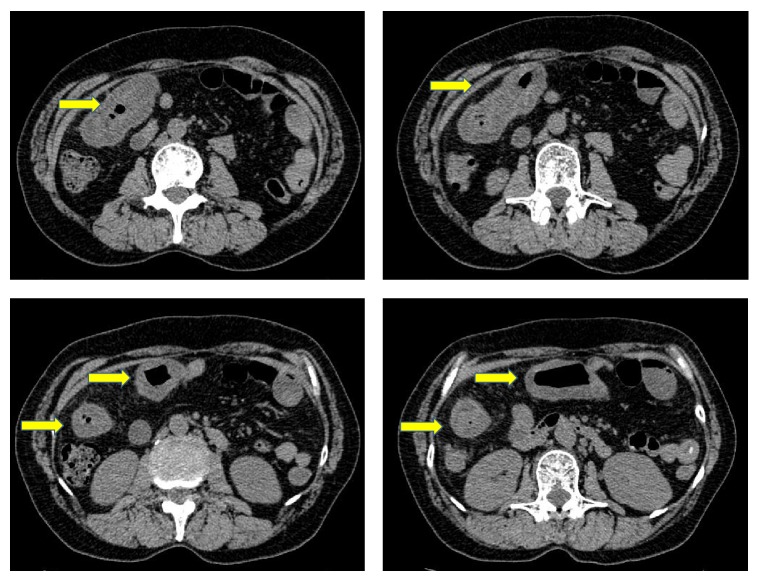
Abdominal computed tomography test revealed the slight edema of the ascending to transverse colon.
